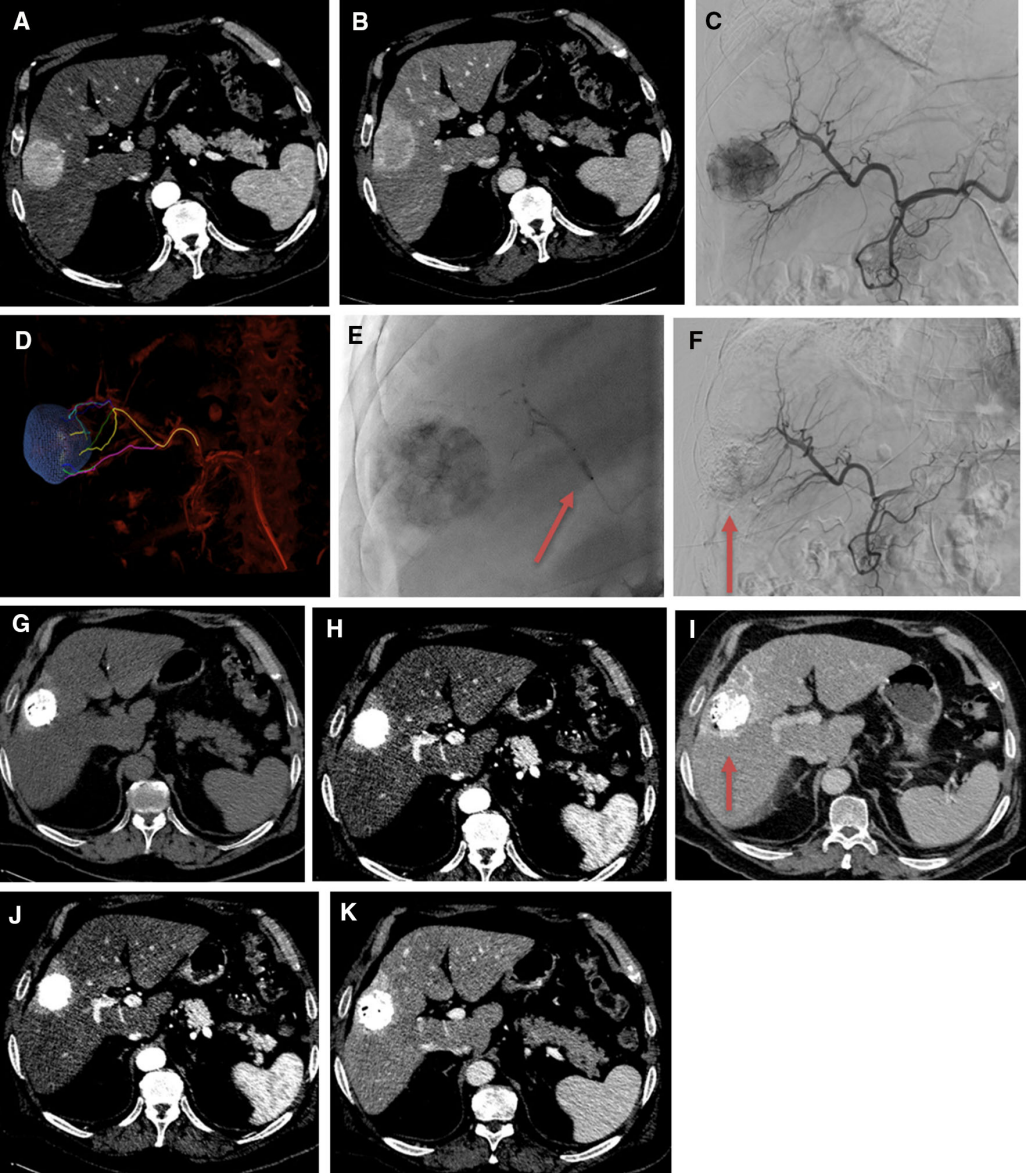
A, B pretreatment computed tomography (CT): a 50 mm HHC is seen in segment V, highly hypervascular in the arterial phase A with a corona enhancement in the portal phase B; C, D pretreatment angiogram and cone beam CT preliminary to balloon inflation and balloon-occluded arterial stump pressure (BOASP) measurement; E, F B-TACE treatment with lipiodol-epirubicin injection during balloon inflation immediately after BOASP measurement (arrow) and final angiographic control F; G, H, I Follow-up CT at 1 month [pre-contrast G, arterial H and venous phases I], showing a size reduction to 43 mm and the dense distribution of Lipiodol, also including the drainage area; J, K Follow-up CT at 6 months: an additional decrease in size to 35 mm, no viable tumour
